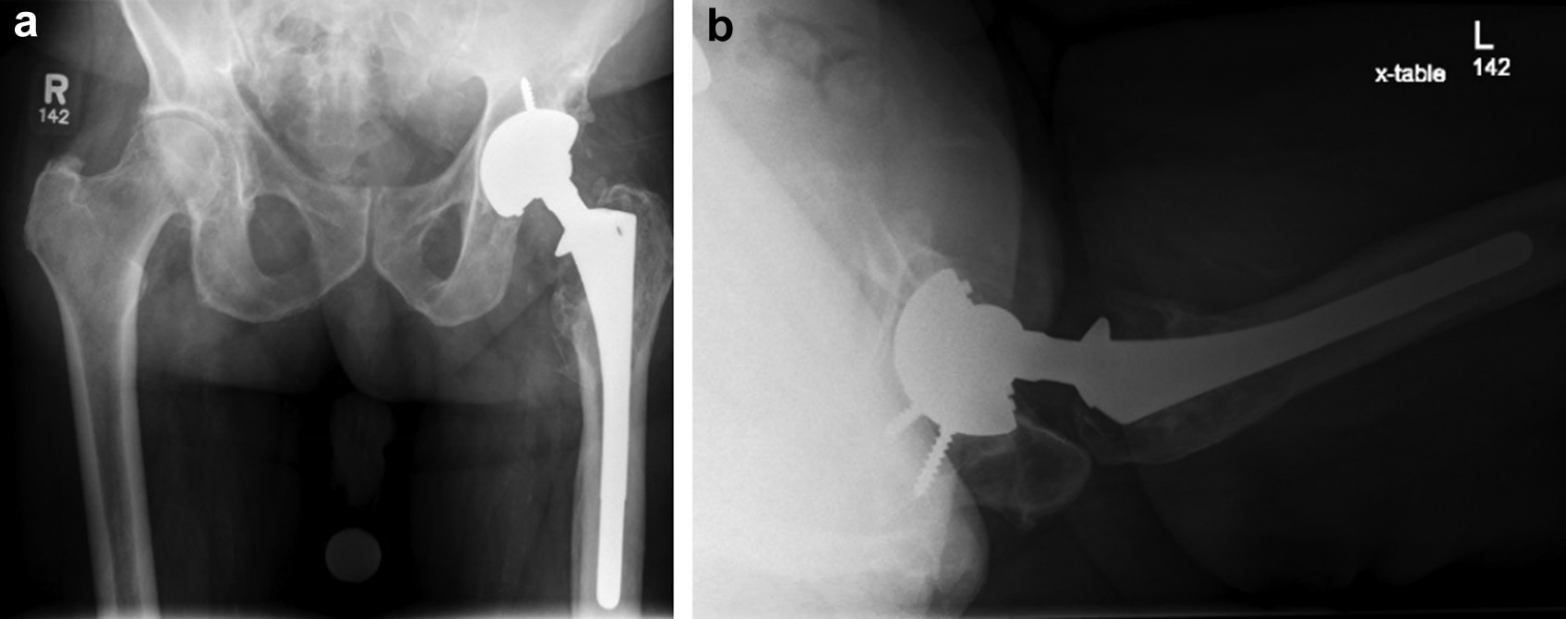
Anteroposterior (AP) pelvis (a) and lateral hip radiograph (b) showing left total hip replacement with extensively ingrown femoral stem, proximal femoral osteolysis, and well-fixed, noncemented acetabular component with minimal eccentricity.
